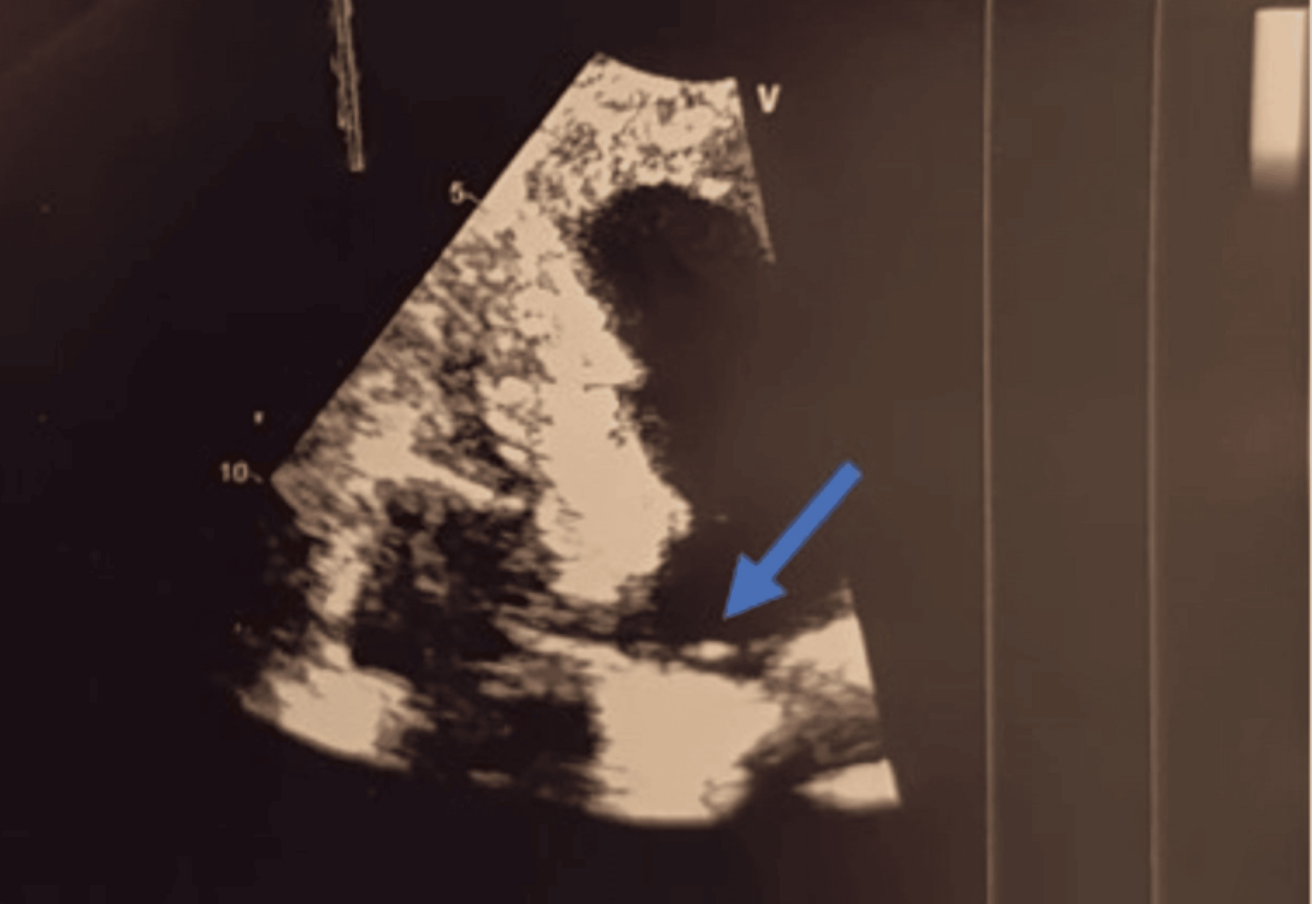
Echocardiogram image showing a non-dilated, non-hypertrophied left ventricle (LV), with an element at the base of the right ventricle (RV) compatible with a tip of an ICD lead (blue arrow) ICD: implantable cardioverter defibrillator
